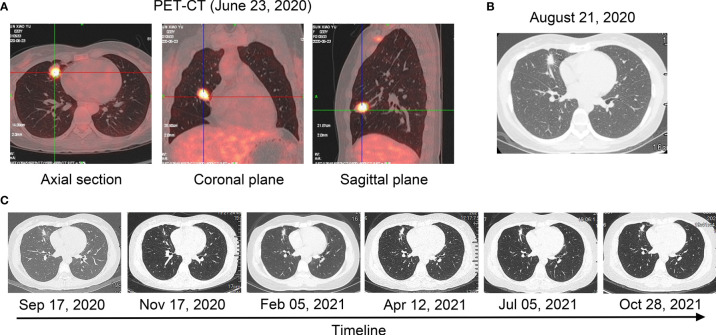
Radiological examinations during the treatment course. (A) PET/CT scan before treatment (23 June 2020). (B) A computer tomography scan after treatment with crizotinib for 6 weeks (21 August 2020). (C) A computer tomography scan after treatment with crizotinib from 17 September 2020 to 28 October 2021.
